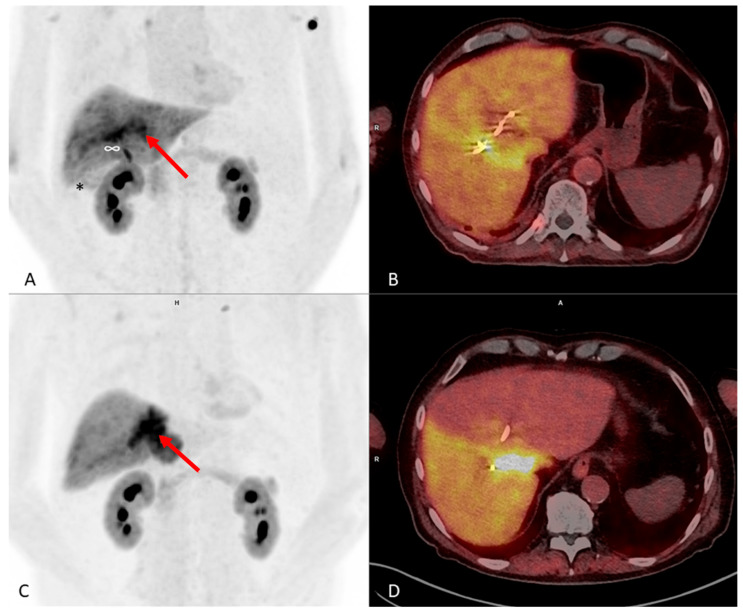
(A,B) MIP and hybrid PET/CT scan slice from June 2024 using [^68^Ga]Ga-FAP-2286: the red arrow marks the primary tumor lesion with a decreasing size and tracer uptake as compared to (C,D) after change of chemotherapy to FOLFOX. SUV_max_ 15.6; SUV_peak_ 13.6. (C,D) MIP and hybrid PET/CT scan slice from March 2024 using [^68^Ga]Ga-FAP-2286: the red arrow marks the primary tumor lesion with an increasing size and tracer uptake at this time point after chemotherapy with cisplatin, gemcitabine, and durvalumab. SUV_max_ 24.6; SUV_peak_ 20.5. Please note a new uptake in image A, which has been interpreted as diffusely reactive after acute cholangitis with cholezystitis (*) that has been treated with antibiotics at the time point of the PET scan. Additionally, there is a new uptake at the proximal common bile duct (∞), as well as diffuse linear-shaped uptake along the central bile ducts and a change of diffuse FAP expression in the liver parenchyma, considered as reaction to the inflammatory process.
